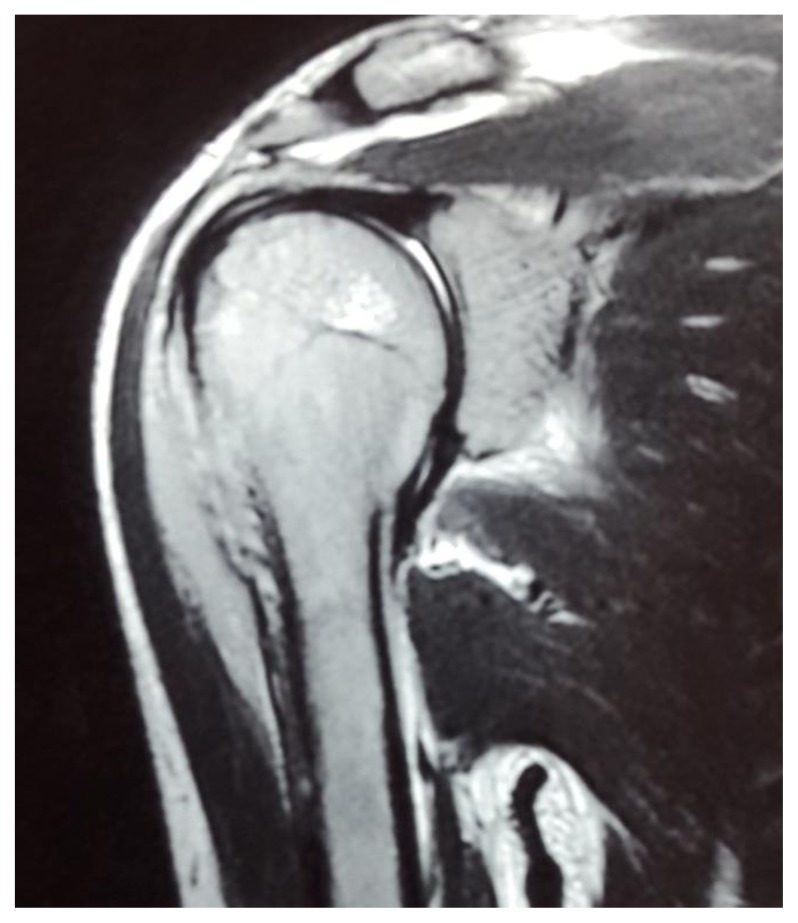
Magnetic resonance imaging showed focal cortical lytic lesion in the head of right humerus and greater tuberocity
